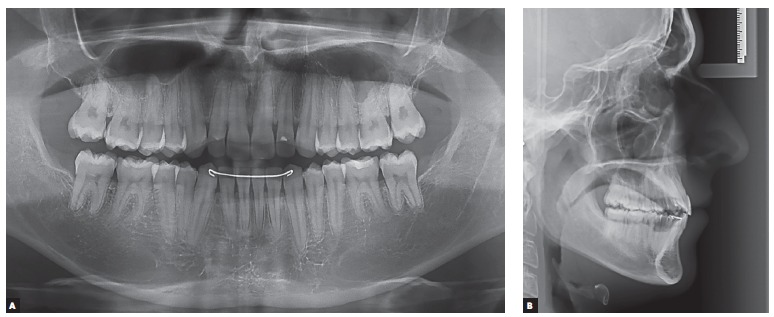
Radiographic follow-up two years after orthodontic treatment completion.
